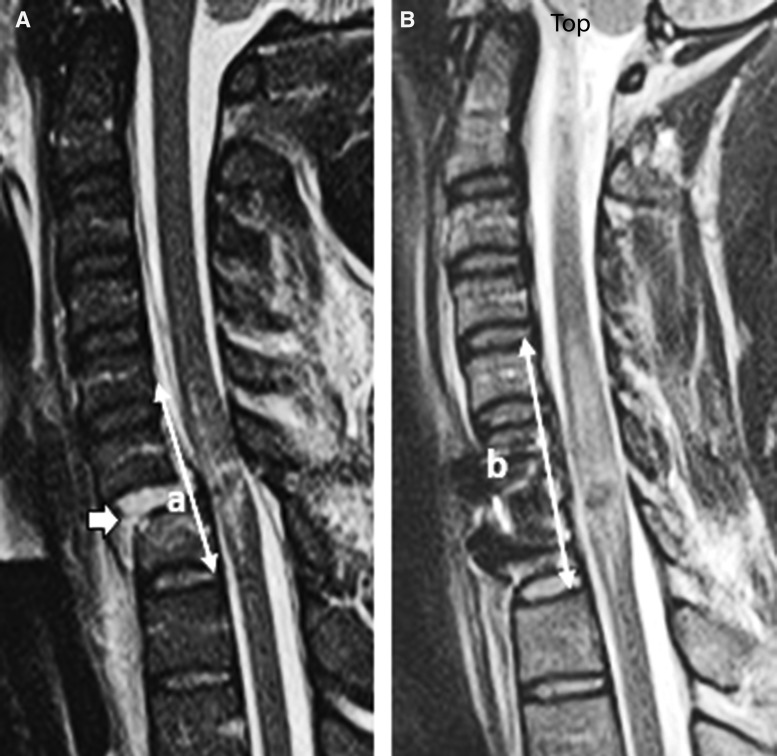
Pre-operative (A) and post-operative (B) midsagittal magnetic resonance images indicating measured intramedullary lesion length a and b.
